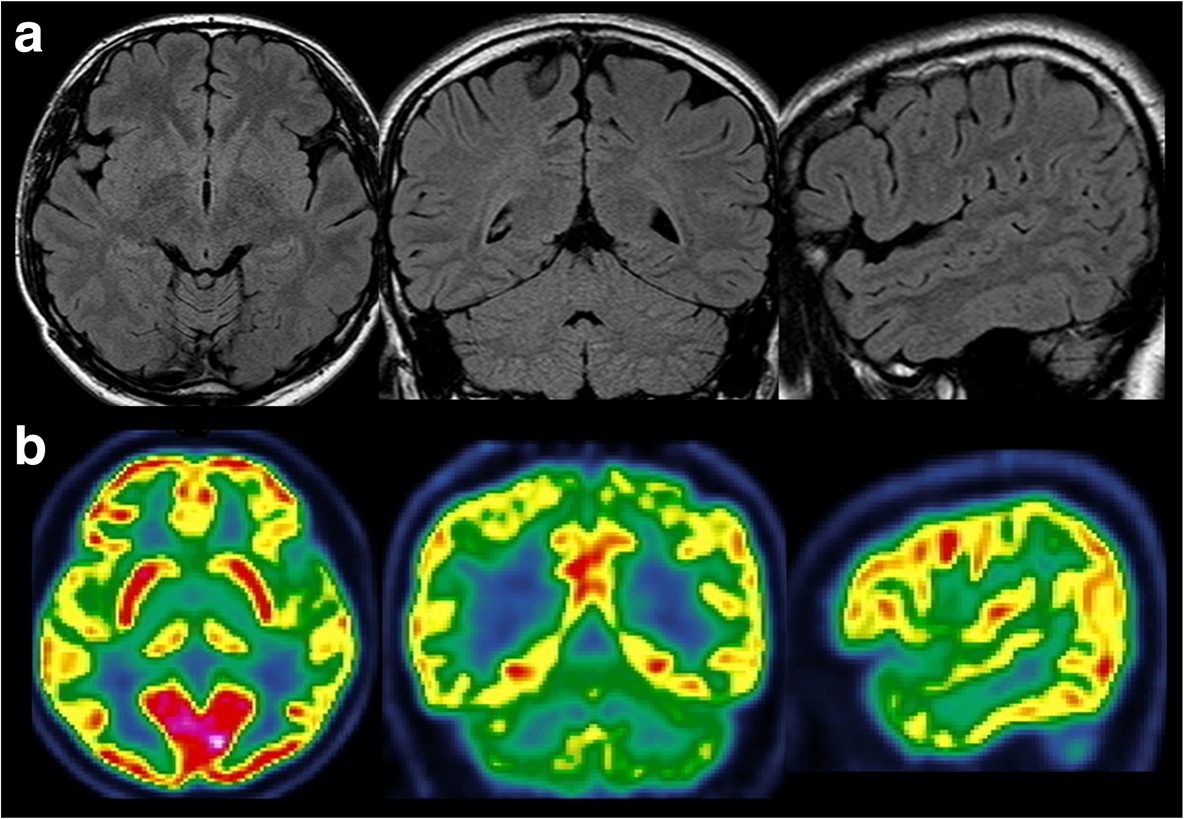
a and b showed the Brain MRI and FDG-PET of Case-1. No evaluable changes were identified for localization of epileptogenic lesion
